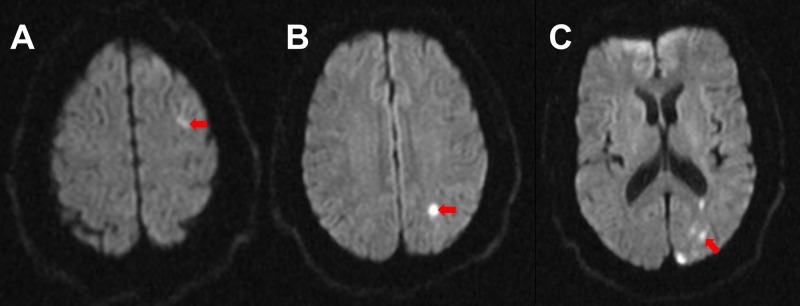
Brain magnetic resonance imaging. Diffusion-weighted magnetic resonance imaging of the brain revealed increase in signal intensity (red arrows) within left frontal (A), temporal (B), and occipital lobes (C).
